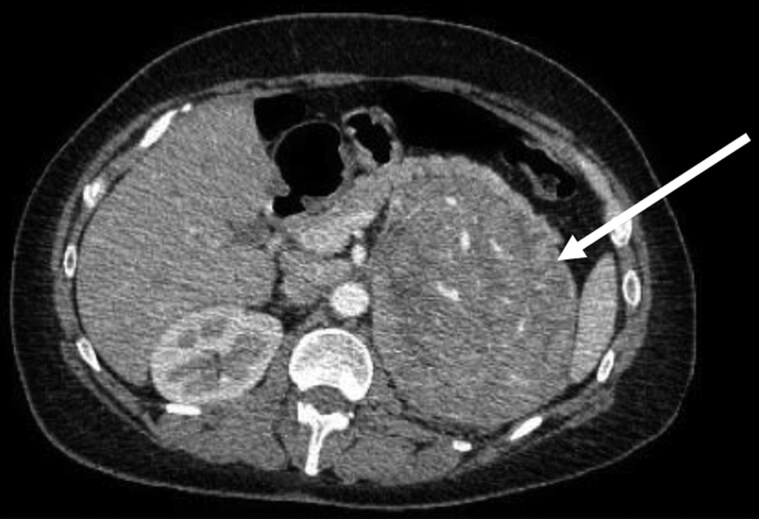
CT adrenals, axial view, showing large left-sided adrenal lesion.
